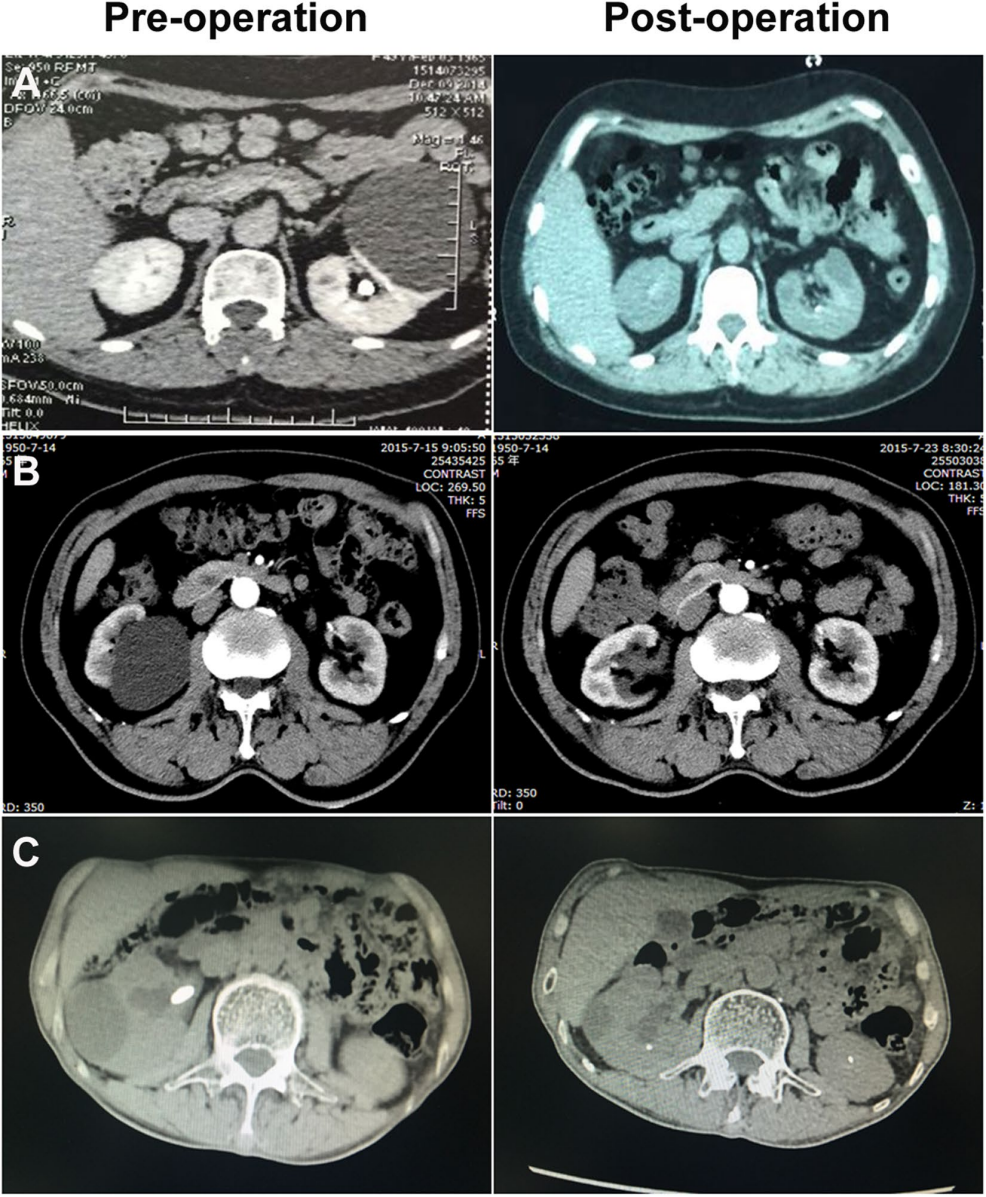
Preoperative and postoperative computed tomography (CT) for patients with peripheral cysts (A), patients with peripelvic cysts (B) and patients with renal cysts complicated with ipsilateral renal calculi (C).
